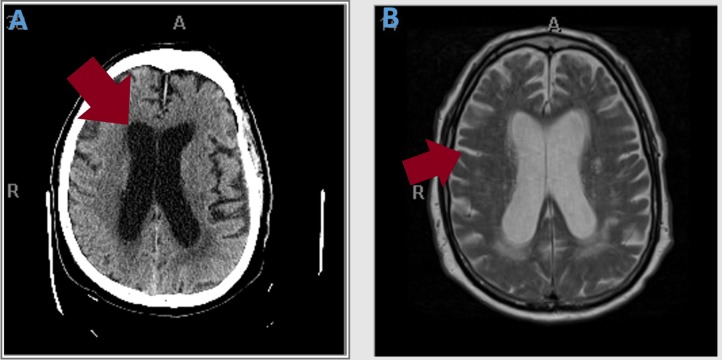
A) Computed tomography scan of the head without contrast (arrow shows ventriculomegaly) and B) Magnetic resonance imaging brain with contrast (arrow shows cerbral atrophy and loss of sulci and gyri)
